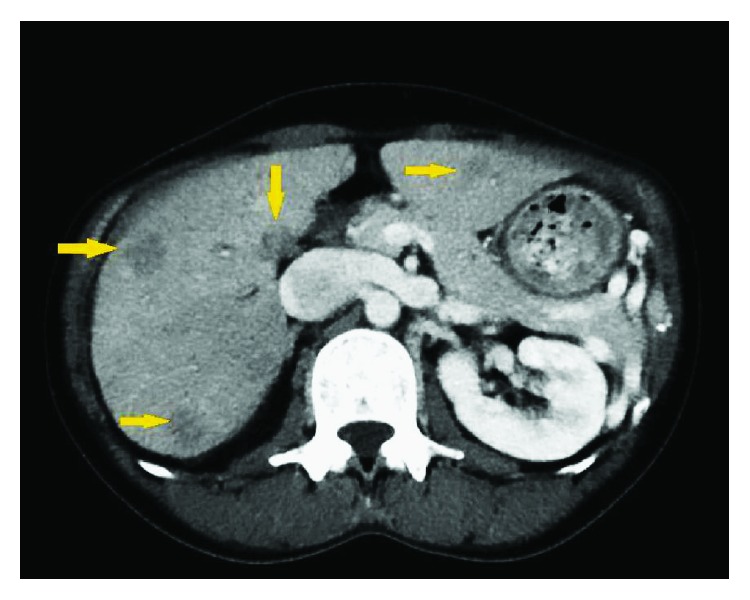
CT scan showing some of the multiple ill-defined liver lesions concerning for metastatic disease in setting of a primary SBO.
